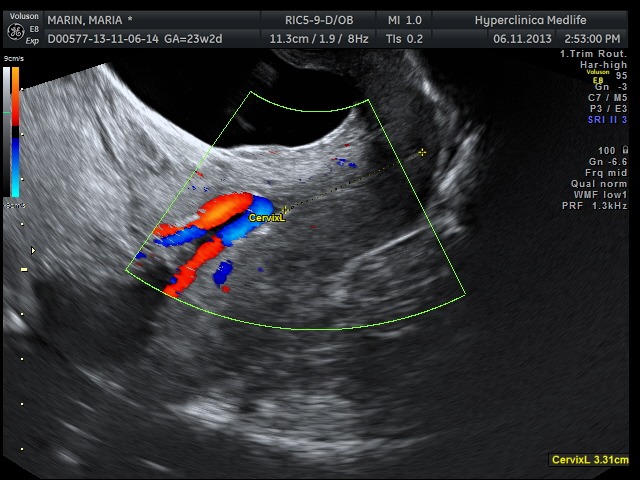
Case 1: measurement of the cervical length by color Doppler transvaginal examination; vessel with a fixed position, which crosses the internal cervical os
